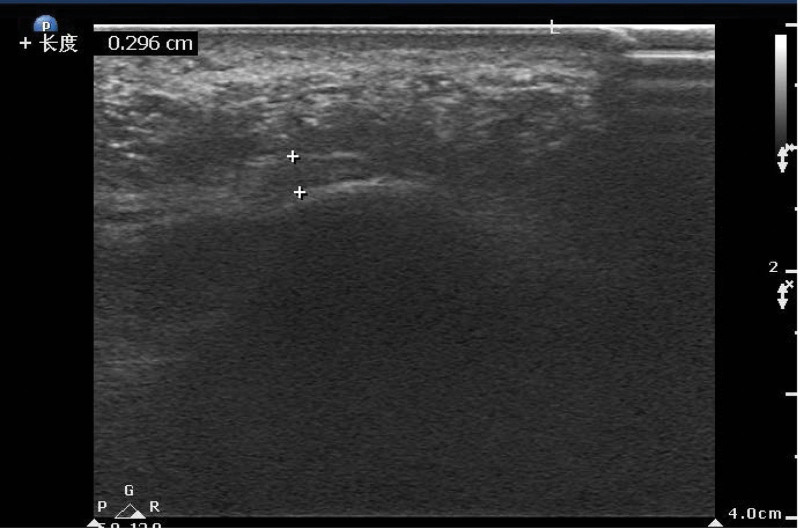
Normal plantar fascia in 2-dimensional ultrasonic longitudinal section was thin band with medium-high echo, and uniform parallel line with high echo, about 0.30 cm in longitudinal section.
